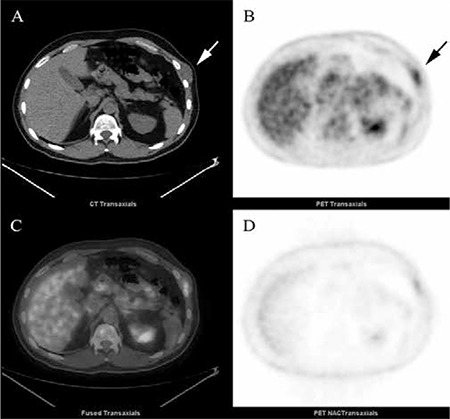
Transaxial (a) CT, (b) PET, (c) PET/CT fusion and (d) Non-attennuation corrected (NAC) PET images show a mildly hypermetabolic ovoid mass lesion (SUVmax: 3.1) in the left 7th intercostal space that is slightly hypodense compared to chest wall muscle (arrows) on non-contrast CT and 32x21 mm in size.
